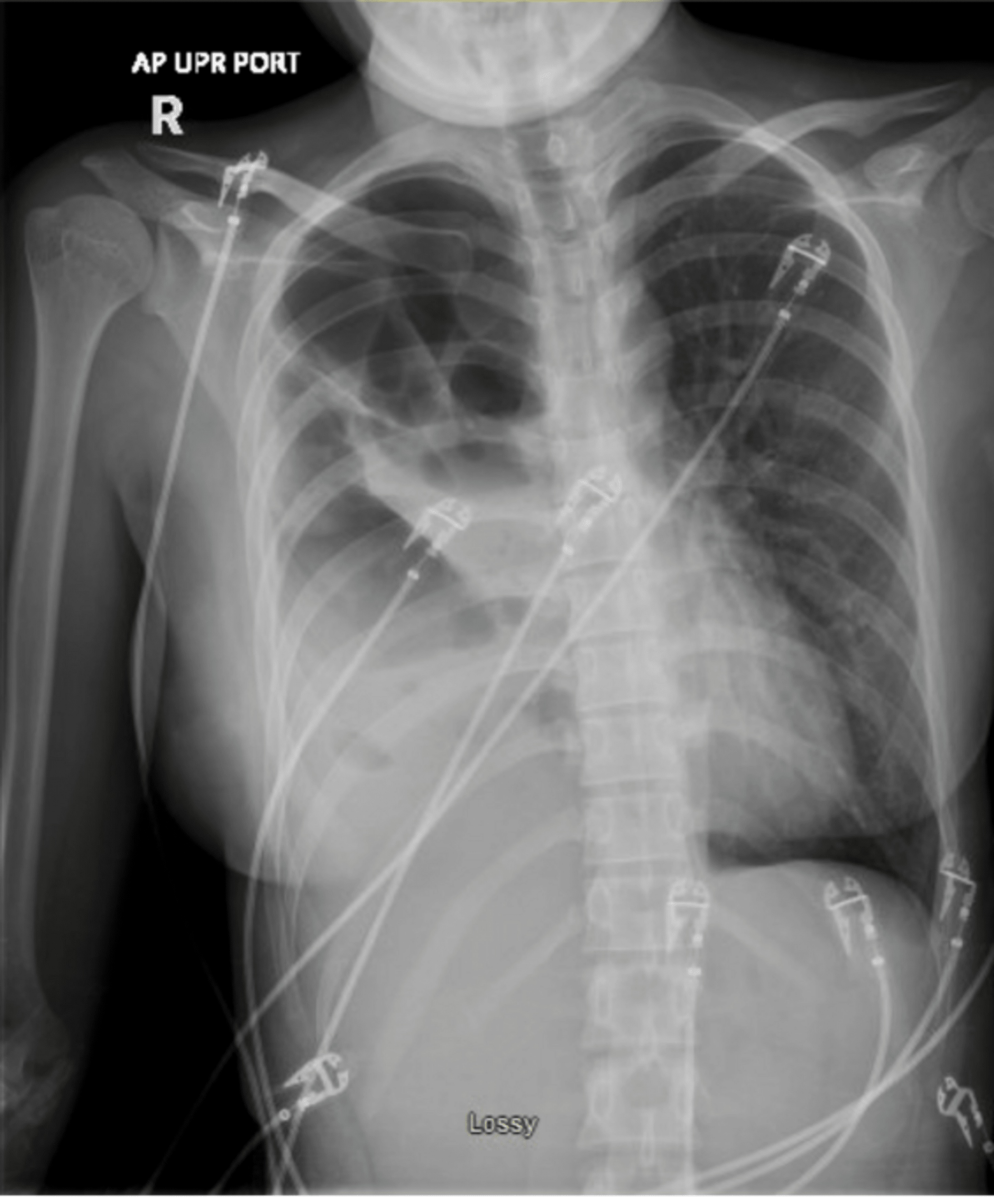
Chest X-ray showing a right-sided pleural effusion with an associated trapped lung.
